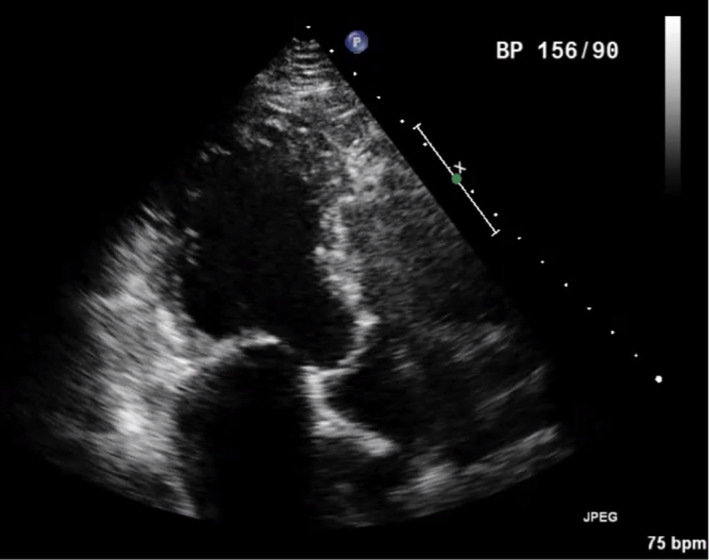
Postoperative Transesophageal Echocardiogram showing absence of LAA after ligation
